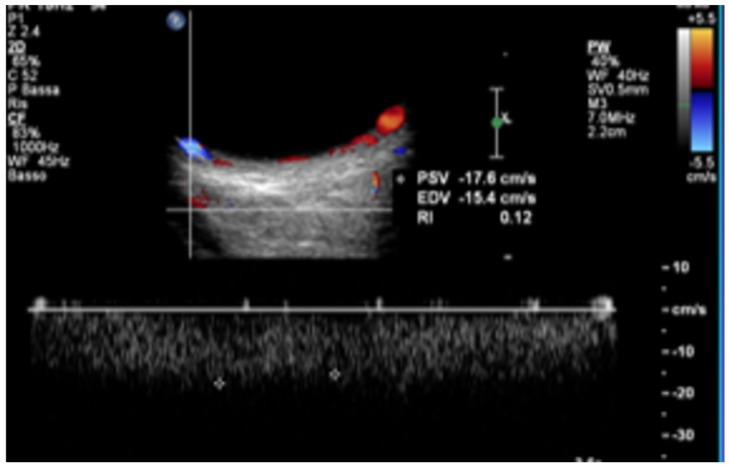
Vortex veins, depicted in blue, course parallel to the ocular globe and are often perpendicular to the ultrasonographic beam. As a result, their Doppler signal may be more difficult to record.
